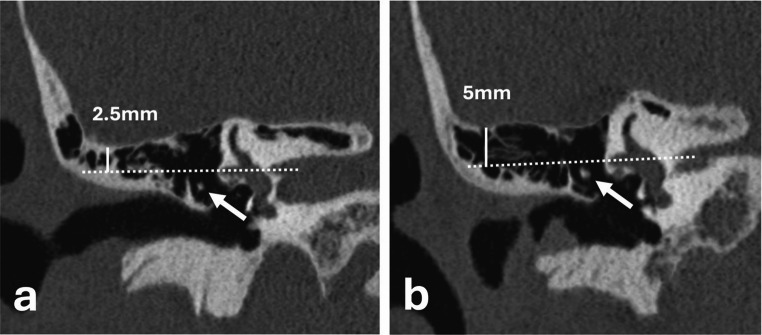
Height of the tegmen tympani. Coronal CT showing measurement of tegmen height (solid lines) above the axis of the lateral semicircular canal (dashed lines), at the level of incus short process (solid arrows). (a) Low-lying tegmen is associated with increased surgical difficulty and risk of dural injury. (b) CT of another patient with normal tegmen height for comparison
